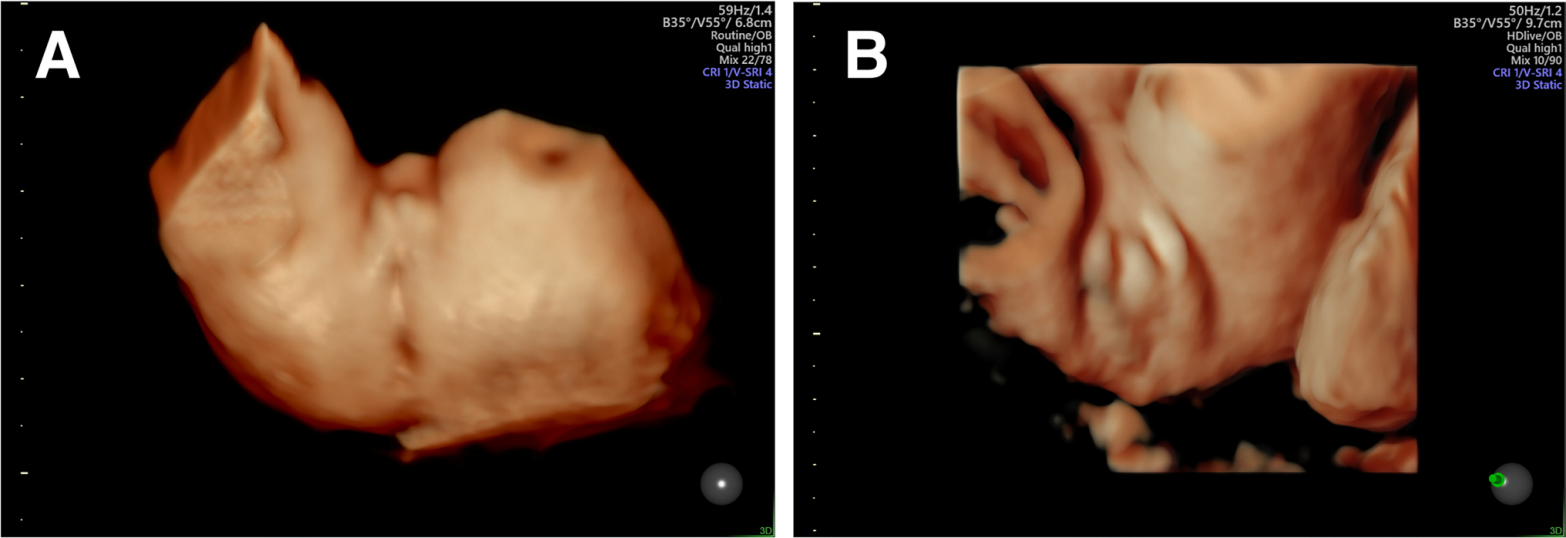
3D sonography of the external female fetal genitalia in a 21 + 0 and b 32 + 2 weeks of gestation
